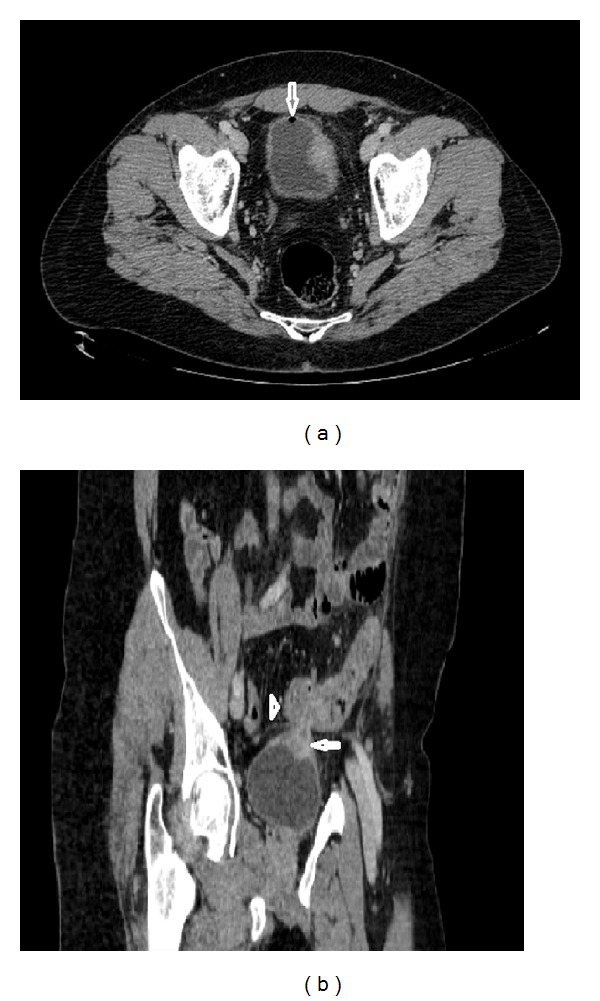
Colovesical fistula: axial image of contrast enhanced CT of the abdomen and pelvis demonstrates air in the bladder (arrow) and thickened left bladder wall (a); sagittal image shows bladder wall thickening (arrow) adjacent to a loop of thickened sigmoid colon (arrow head) (b).
